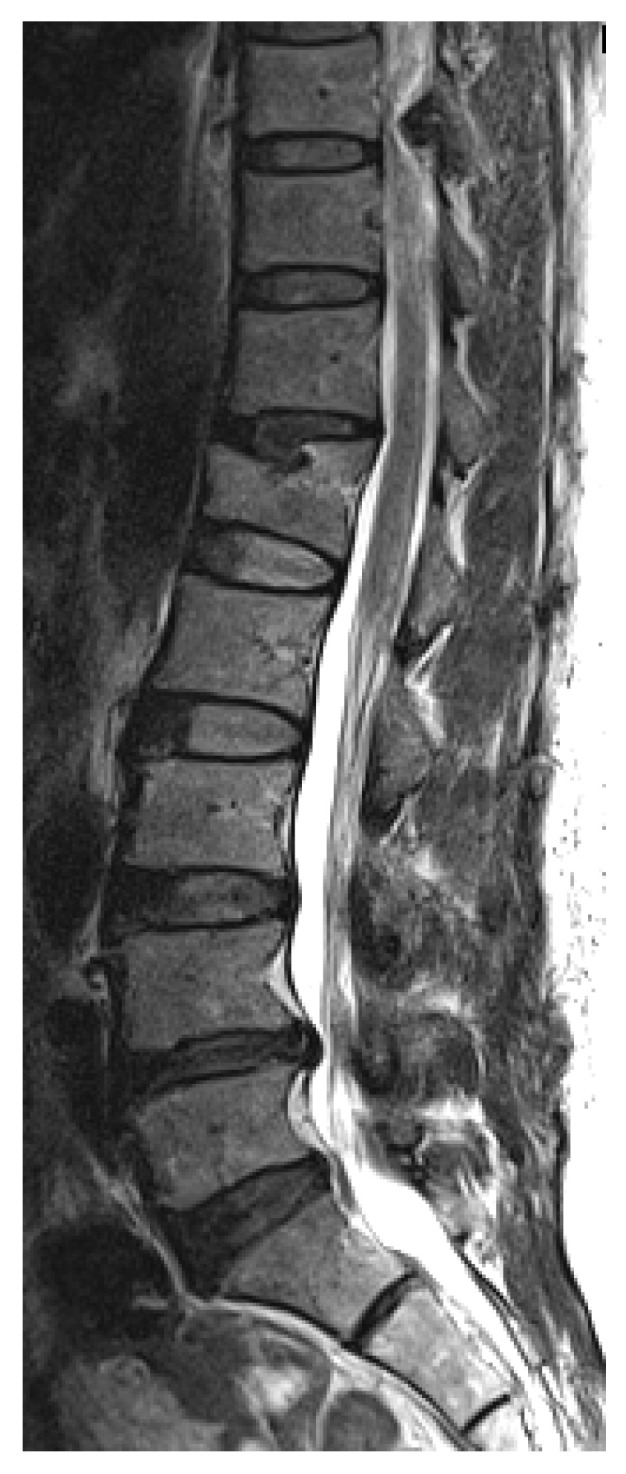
Representative MRI appearance of thoracic ossification of the ligamentum flavum (OLF). Sagittal T2-weighted image demonstrating focal low-signal ossification at the T10-11 level with associated dural indentation.
